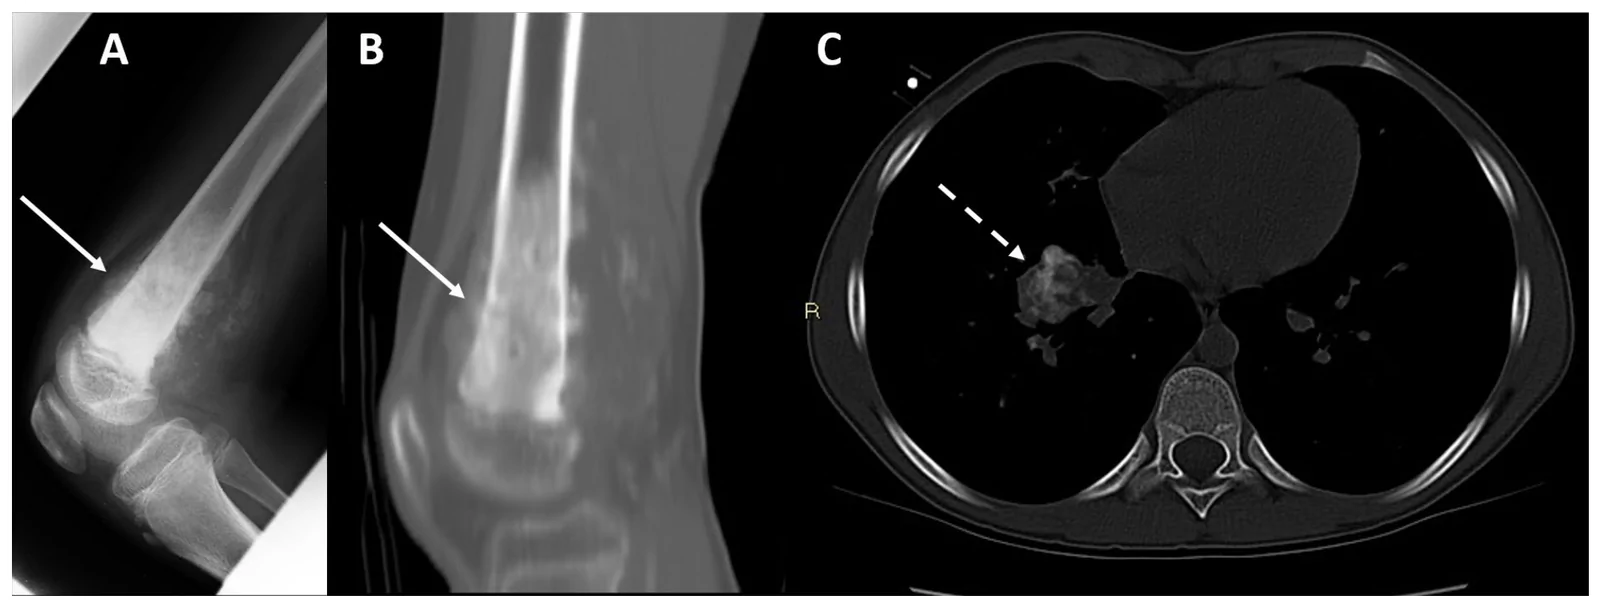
An 11-year-old boy with high-grade osteoblastic osteosarcoma of the right distal femur. Panels (A) (conventional radiology) and (B) (CT with sagittal reconstruction) show a purely sclerotic radiologic pattern; Panel (C) (baseline CT with bone window) shows lung metastases with diffuse calcifications. Lesions are indicated by arrows.
